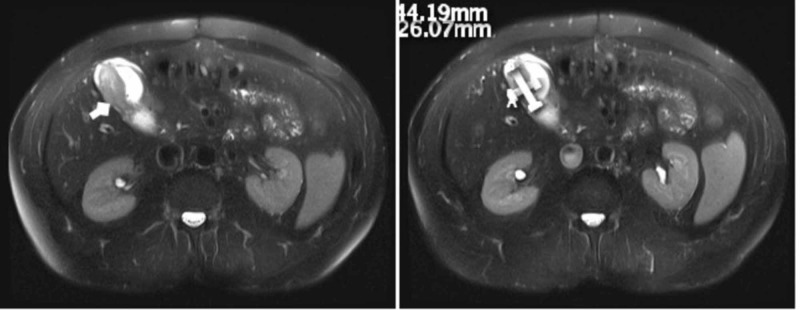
Posterior gallbladder fundus mass (white arrow) on MRI, initially suspected for GIST recurrence versus new malignancy. MRI: Magnetic Resonance Imaging, GIST: Gastrointestinal Stromal Tumor
